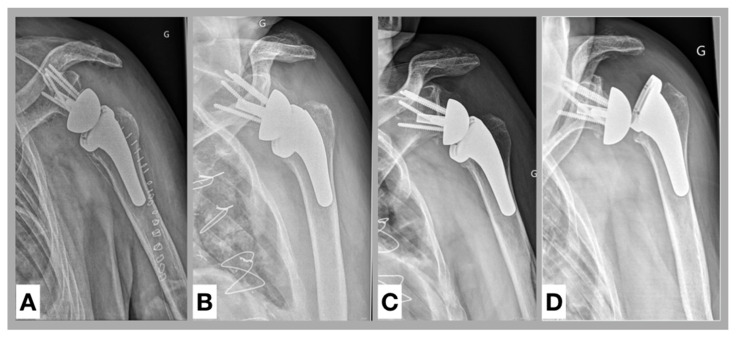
Complication following subsidence. Anteroposterior radiographs of a left shoulder. (A). Postoperative, (B). 3 months follow-up, (C). 6 months follow-up, (D). 9 months follow-up: Due to subsidence a dissociation between the tray and the stem (Morse taper) occurred.
